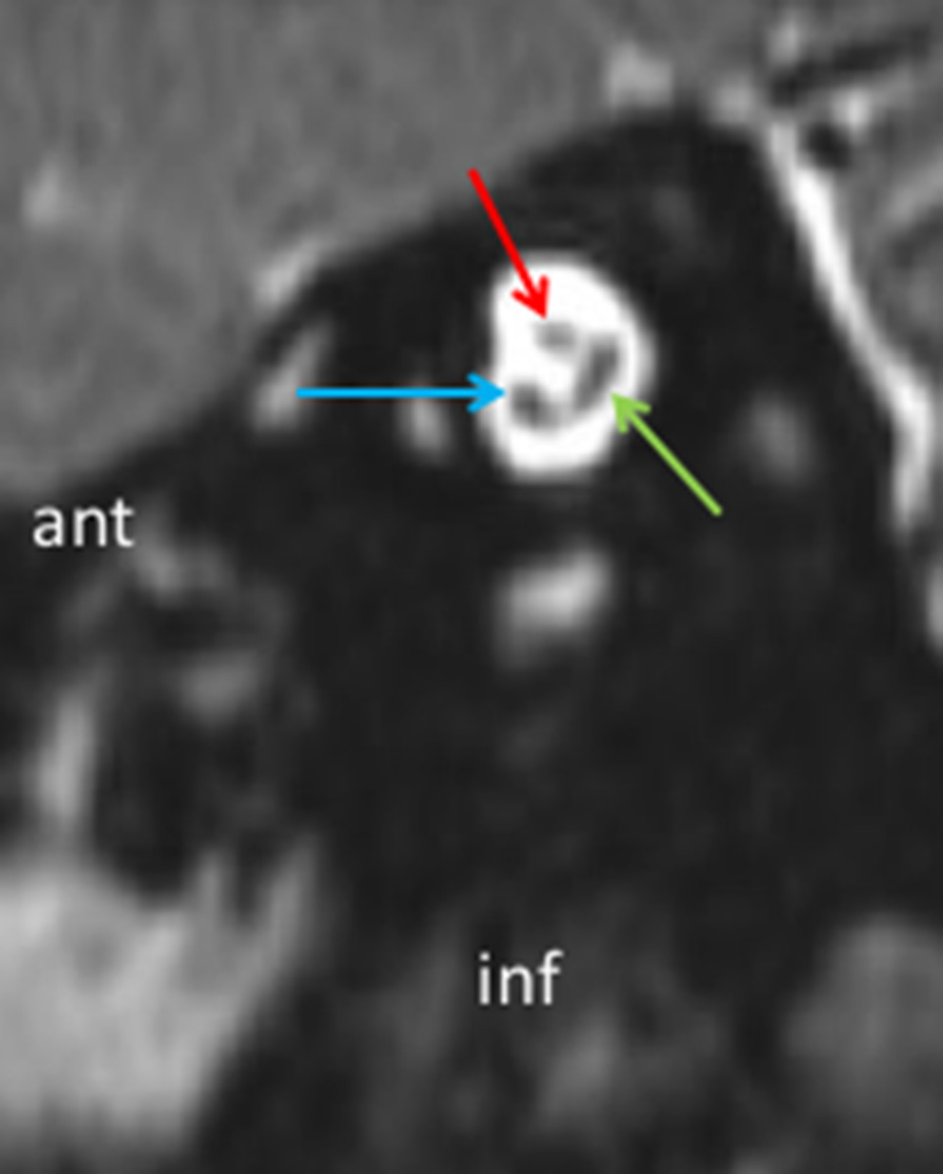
Parasagittal MRI reformat perpendicular to the IAC showing VIIth nerve (red arrow), cochlear nerve (blue arrow) and vestibular nerves (green arrow) with the inferior and superior vestibular branches still unified. IAC, internal auditory canal.
